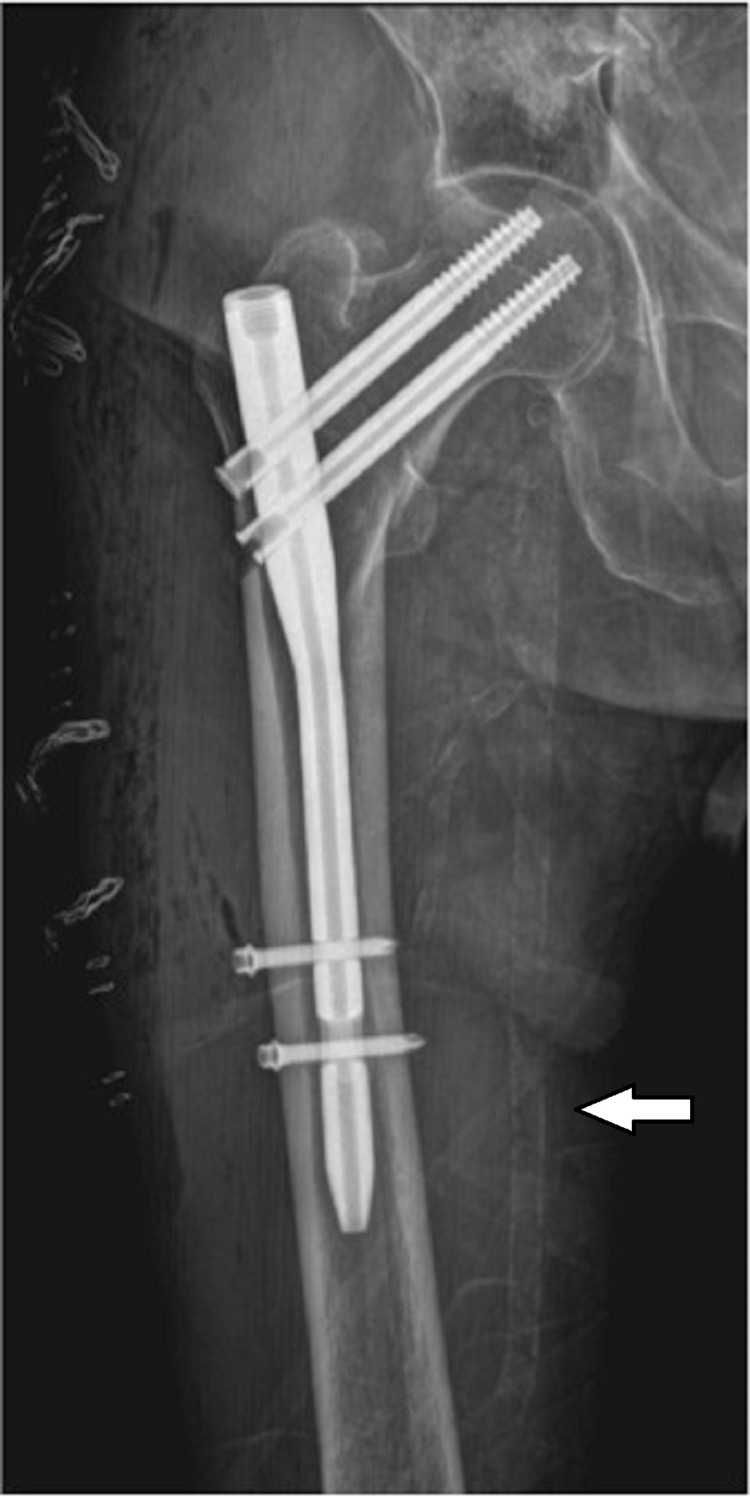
Radiographic image of the medial type femoral artery calcification.
